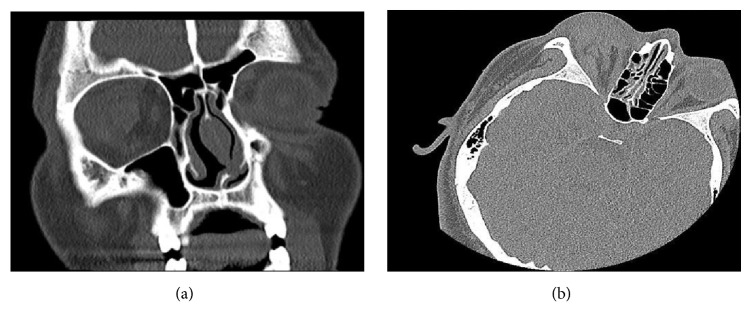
Right globe rupture on nonenhanced CT scan of the orbits.
